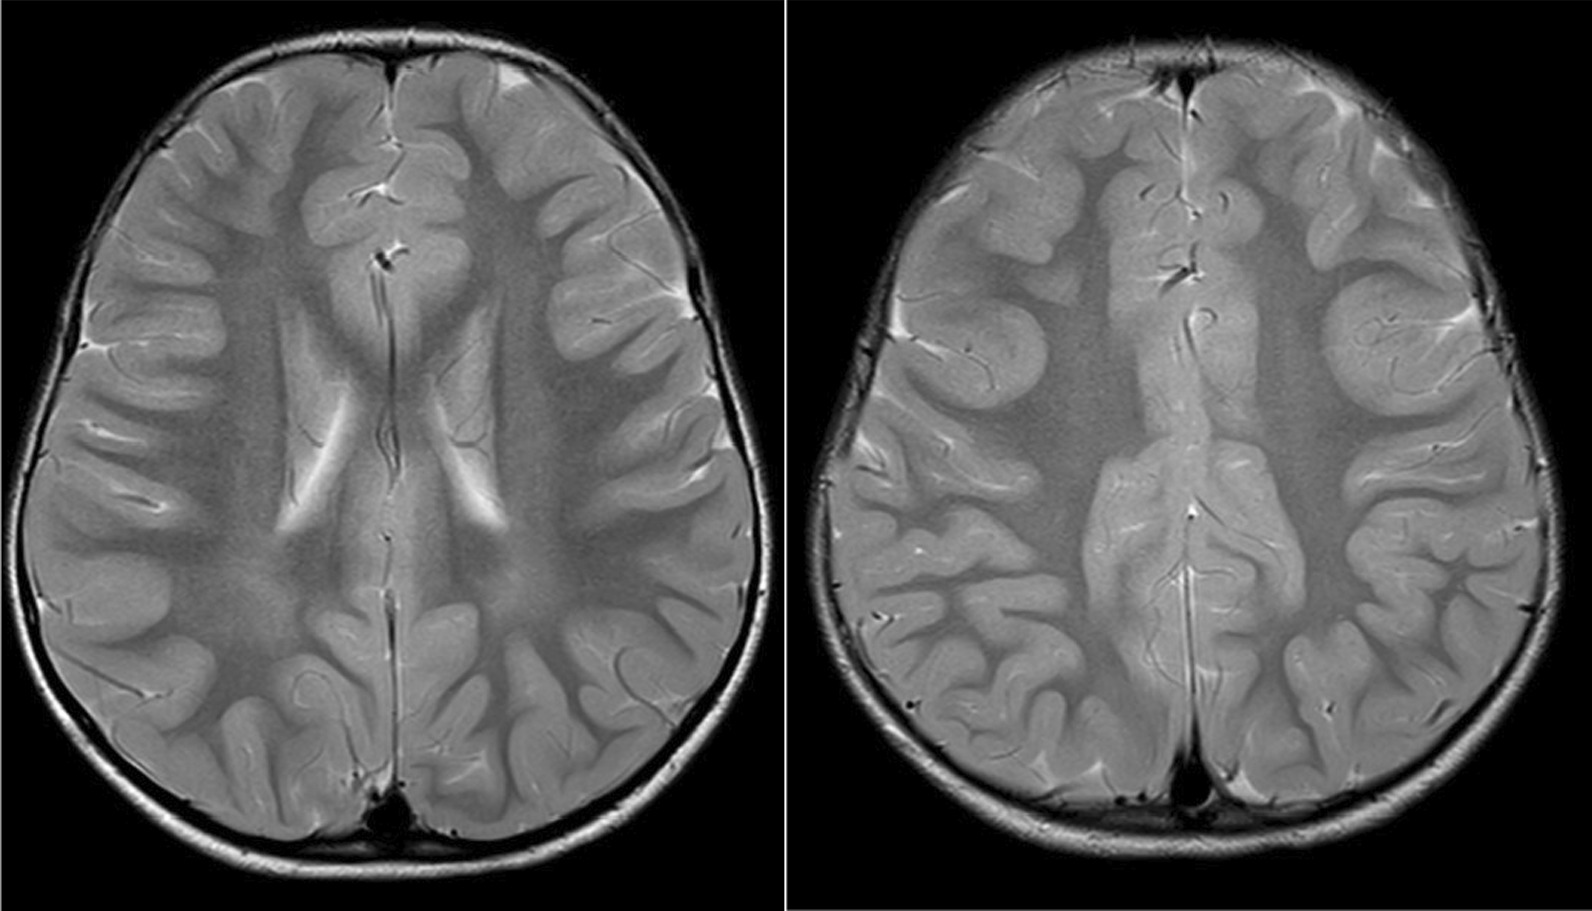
MRI brain of patient 14 taken at 3 years 8 months showing bilateral symmetrical T2 hyperintense changes in the periventricular white matter posteriorly
